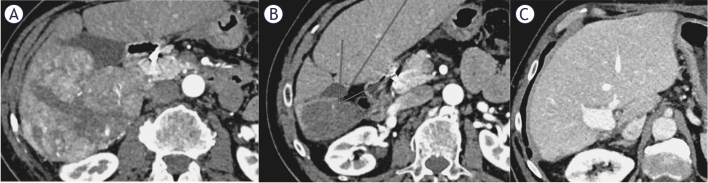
63-year-old male with a 100 mm hepatocellular carcinoma (HCC), chronic hepatitis C (CHV), without cirrhosis, with portal vein tumour thrombosis (Japanese classification Vp4) before treatment (A). Patient received transarterial radioembolization (TARE) and 13 cycles of atezolizumab/bevacizumab, achieving complete response on follow-up CT scan after 14 months (B). Subsequently, surgical resection was performed, with no signs of disease progression (C).
